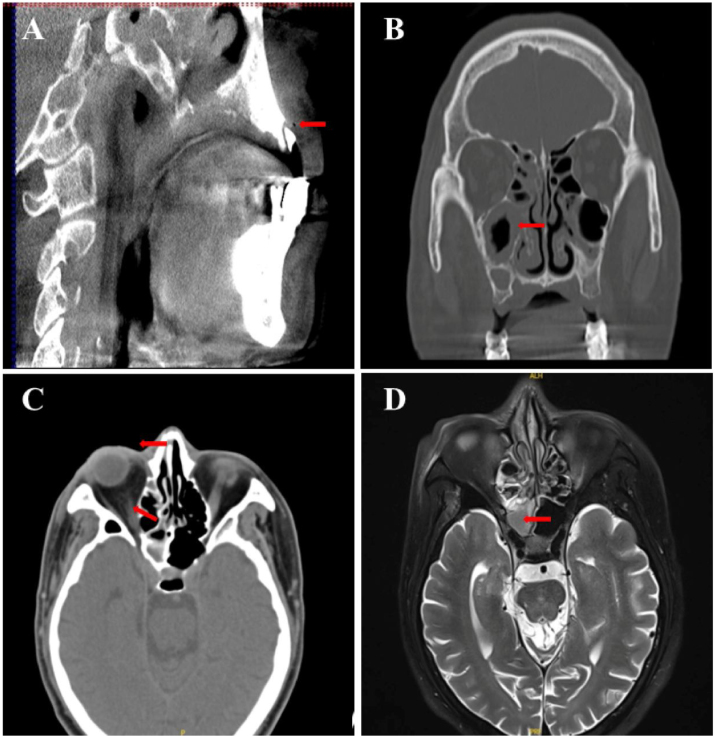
Computed tomography images. (A) Apical periodontitis in the maxillary anterior teeth. (B) Concurrent maxillary and ethmoid sinuses. (C) Right optic perineuritis with proptosis. (D) MRI showing prominent inflammation of the right maxillary and ethmoid sinuses.
